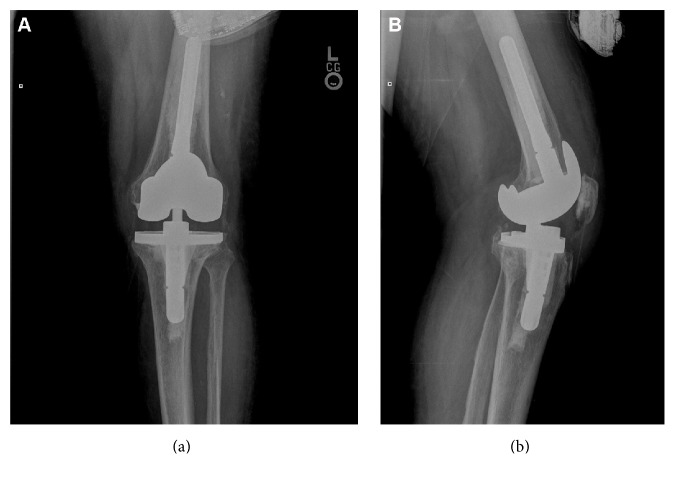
Anterior-posterior (a) and lateral (b) radiographs after second stage of reimplantation of cemented revision left total knee arthroplasty.
